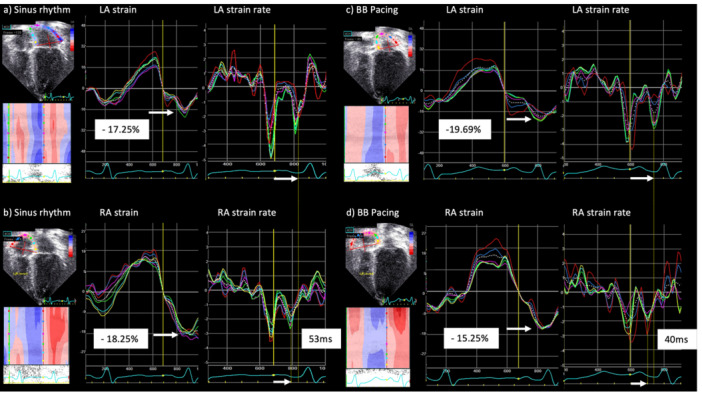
Assessment of mechanical atrial synchrony using speckle tracking echocardiography. Comparison of longitudinal strain and strain rate curves during intrinsic sinus rhythm (a and b) versus BB pacing (c and d). (a and b) The average longitudinal strain was −17.25% for the left atrium (LA) and −18.25% for the right atrium (RA). The interatrial conduction delay (difference in time to negative peak [TTNP] strain rate between LA and RA) was 53 ms. (c and d) BB pacing resulted in improved mechanical resynchronization. The interatrial conduction delay was reduced to 40 ms. LA and RA longitudinal strain were similar to sinus, measuring −19.65% and −15.25% respectively. GS, global strain; LA, left atrium; RA, right atrium; TTNP, time to negative peak.
